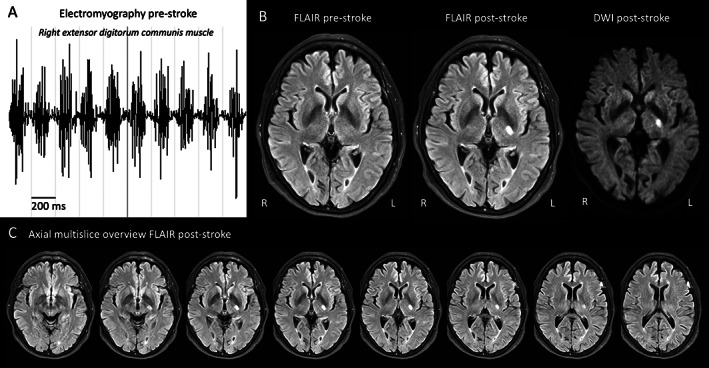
Polymyography and magnetic resonance imaging (MRI) of the brain pre‐ and post‐stroke. (A) Surface electromyography shows tremulous with a frequency between 4.3 and 5.6 Hz of right extensor digitorum communis muscle pre‐stroke. (B) On the left, a T2‐fluid‐attenuated inversion recovery (FLAIR) sequence pre‐stroke shows no abnormalities. Next a FLAIR sequence post‐stroke showing a hyperintensity in the posterior limb of the internal capsule, in the vascular territory of the anterior choroidal artery, which is confirmed on a diffusion‐weighted image (DWI, b = 1000). (C) Axial multislice overview of FLAIR images post‐stroke.
